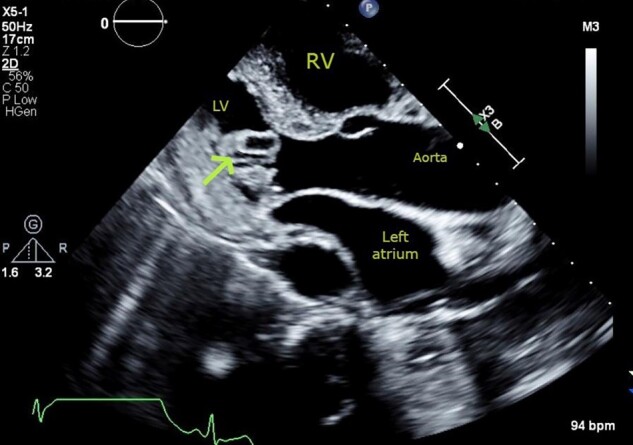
Transthoracic echocardiogram. Parasternal long-axis view showing cystic mass (arrow) with echo-lucent core, protruding into the left ventricular cavity from the basal infero-lateral wall.
